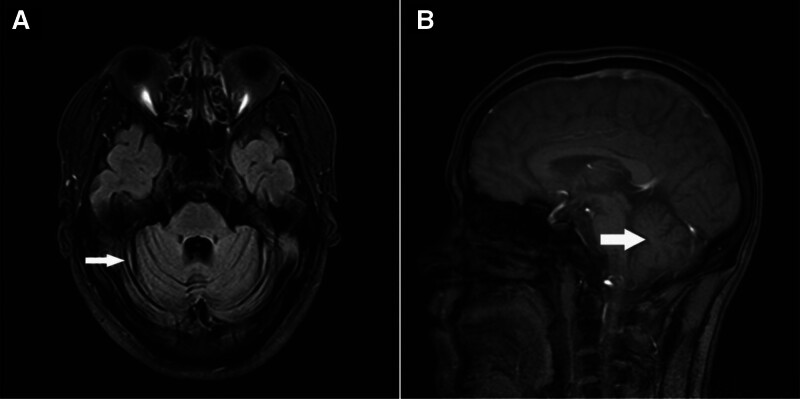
(A and B) Head MRI results of the proband: the brain parenchyma shows no abnormal signals, with a clear demarcation between the gray and white matter. The size and signal of the ventricular system were normal. There was bilateral widening of the cerebellar sulci, whereas the cerebral sulci and cisterns appeared normal. The midline structures were not displaced, and the brainstem contour was well defined. MRI = magnetic resonance imaging.
